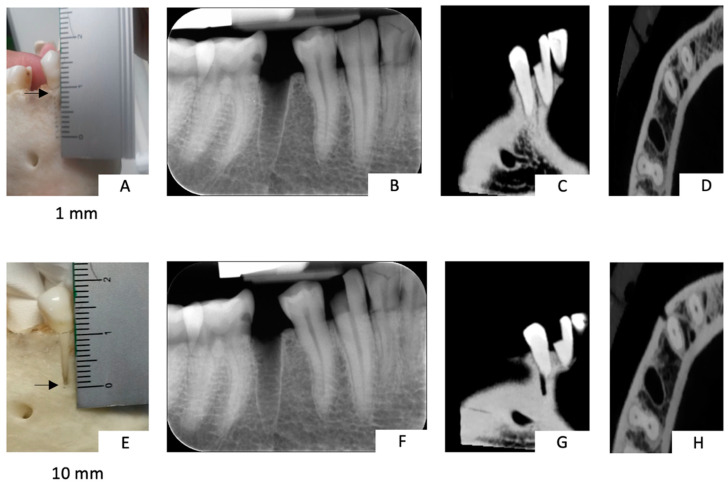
The buccal bone defect was created at the most coronal level of a first premolar in the mandibula and was enlarged apically in steps of 1 mm: (A) buccal defect of 1 mm was created; (B) periapical radiography and (C,D) CBCT of the 1 mm defect; (E) buccal defect of 10 mm created; and (F) periapical radiography and (G,H) CBCT of the 10 mm defect.
